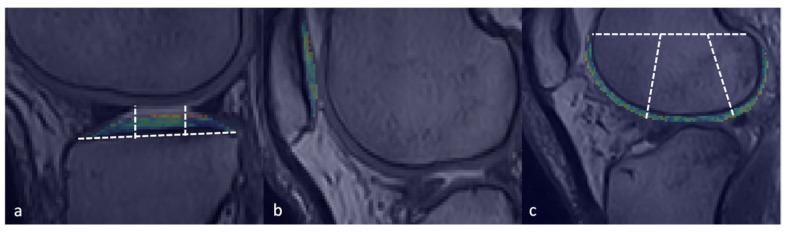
Image analysis according to the Whole-Organ Magnetic Resonance Imaging Score (WORMS) classification. Representative examples of manually drawn regions of interest (ROIs) for T2 relaxation time measurements are shown with dashed lines for the (a) tibial plateau, (b) patella, and (c) femoral condyle.
